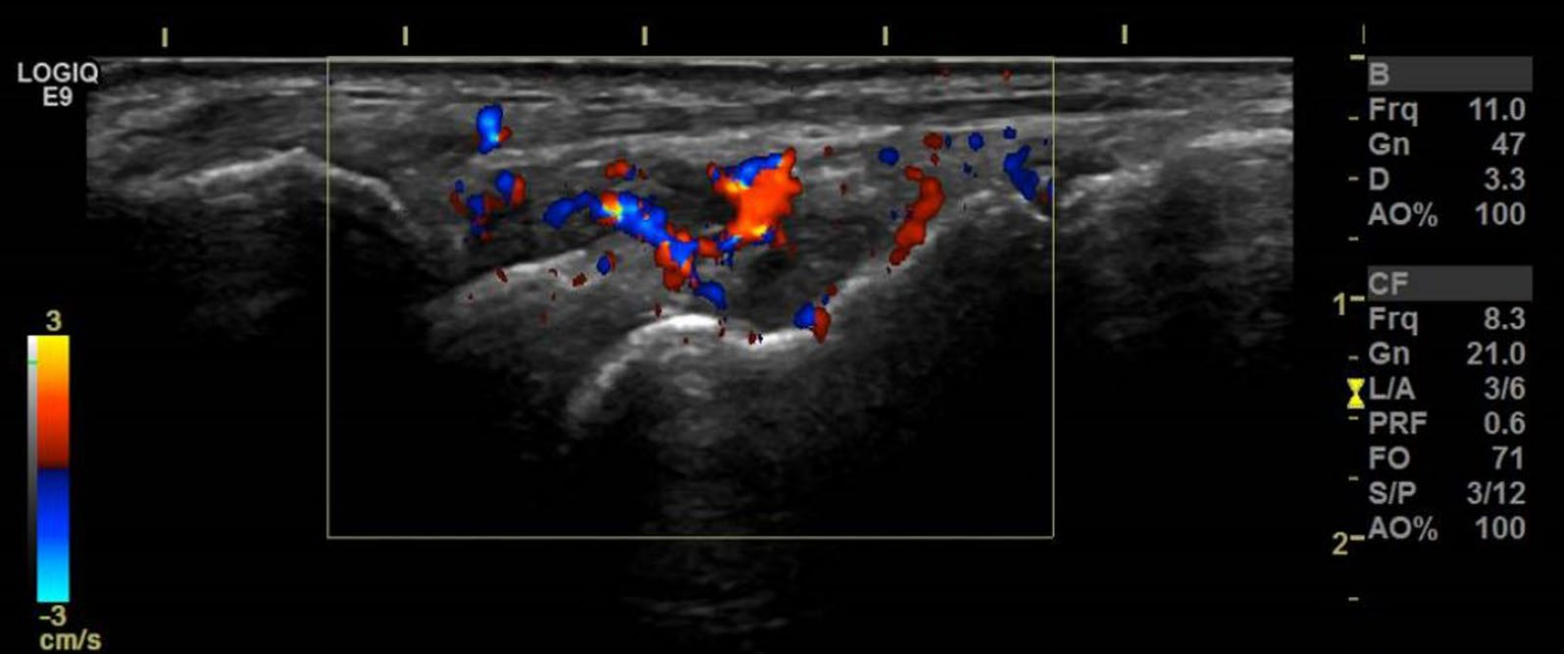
Ultrasound of the right wrist, dorsomedial aspect, with high color Doppler activity
